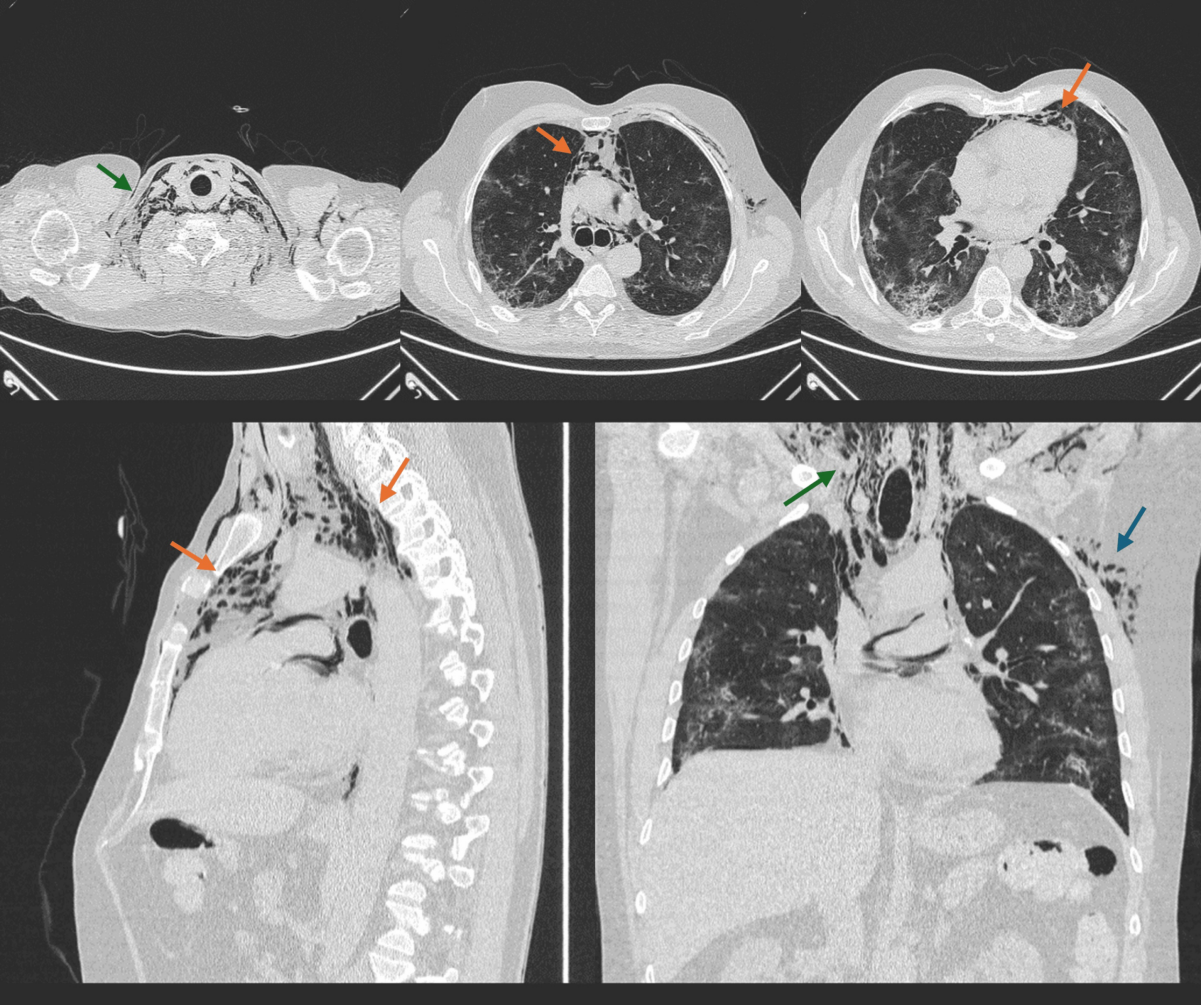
CT scan showing severe pneumomediastinum (orange arrows), and emphysema of the soft tissue of the neck (green arrows) and left lateral chest wall (blue arrow) in a patient with COVID-19 pneumonia.
